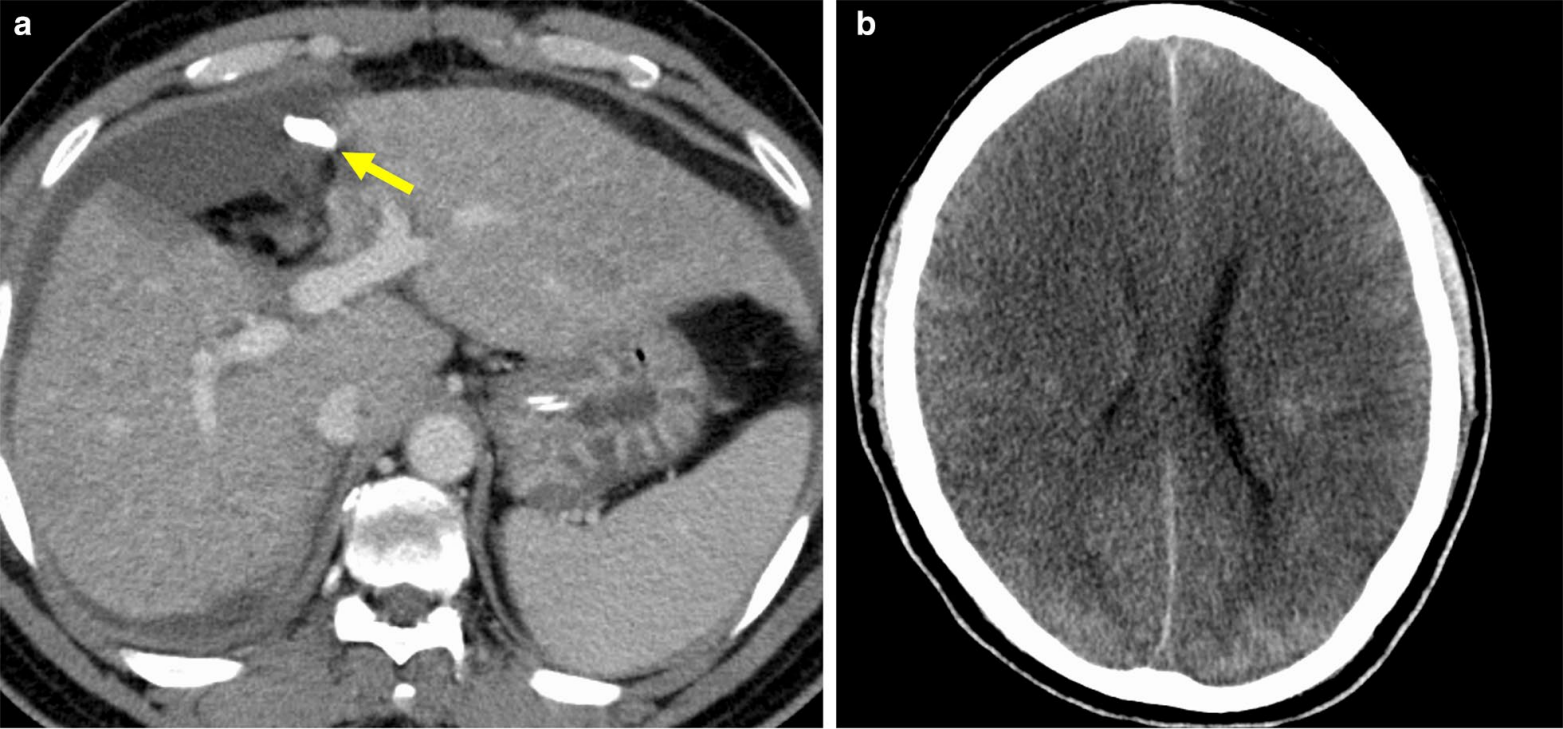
Contrast-enhanced computed tomography image performed on postoperative days 13 (a) and 16 (b). Liver parenchyma showed a slight inhomogeneous enhancement without apparent occlusion of the hepatic arteries and portal vein. The arrow indicates an abdominal drain (a). Diffuse cerebral edema and impaired enhancement of cerebral parenchyma were noted (b)
